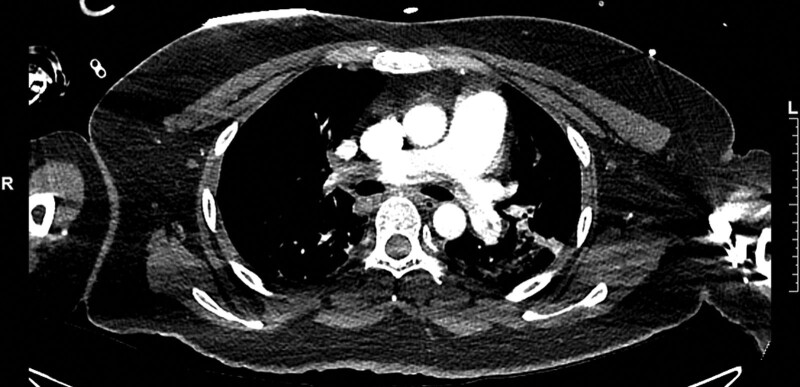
Axial computed tomography image showing thrombosis in the right main pulmonary artery.
